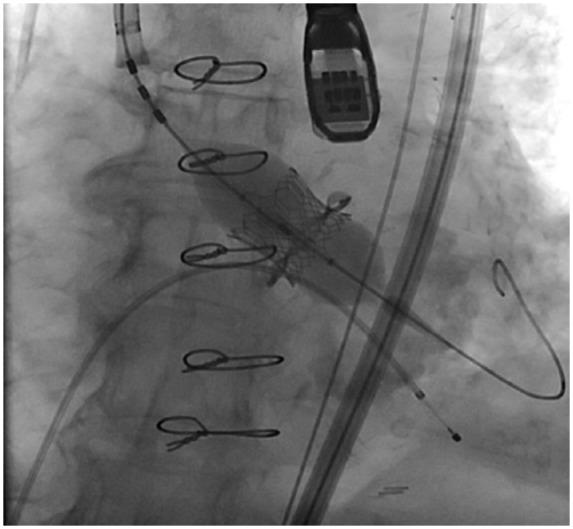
Transesophageal echocardiography guided implantation of a 20-mm Edwards Sapien 3 valve inside a 21-mm Mitroflow bioprosthesis.
